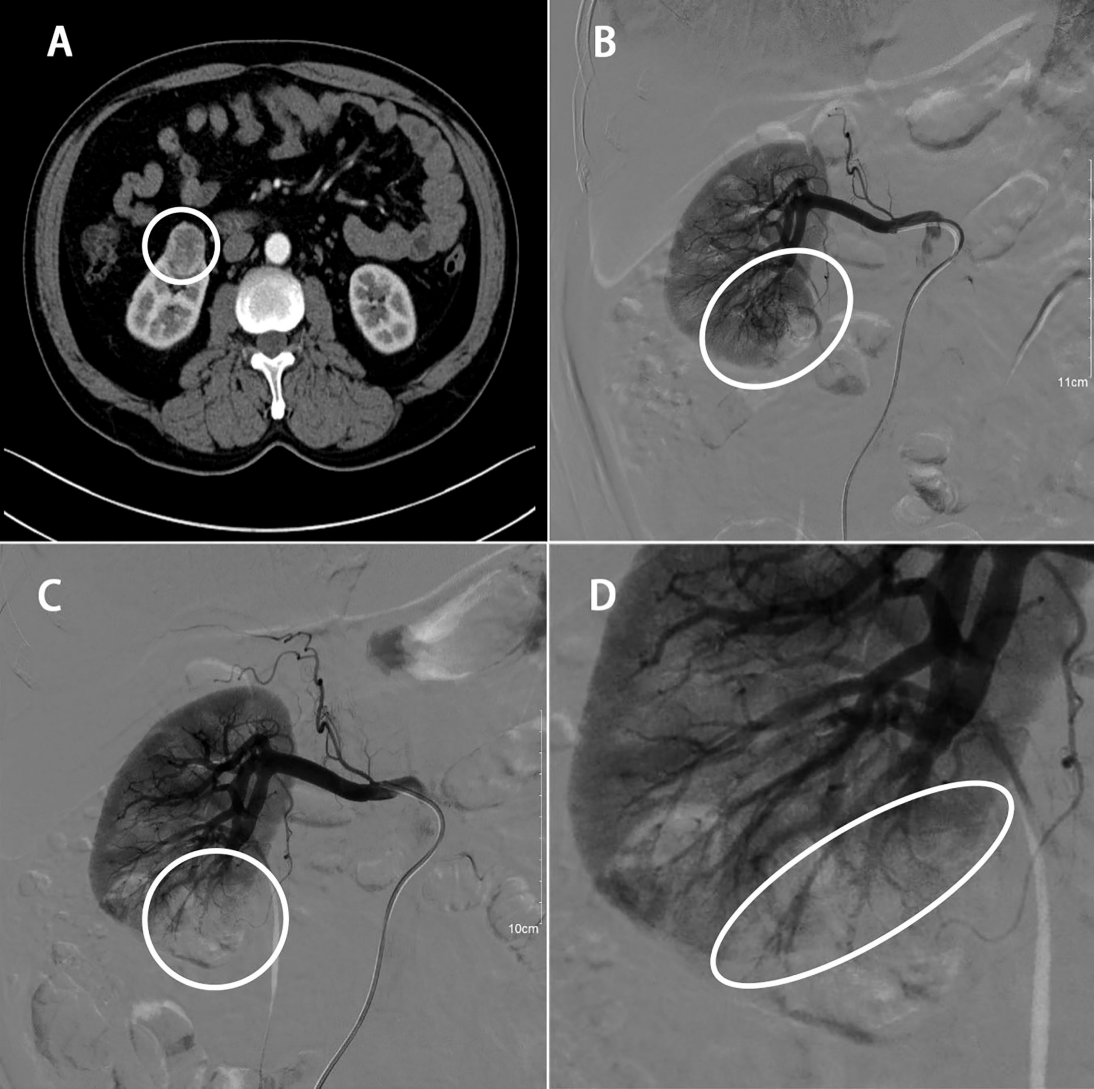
58 years old male with RCC of the right kidney lower pole (40 x 30 x 25 mm). (A) Preoperative enhanced CT scan; (B) renal arteriogram; (C) STE of order artery feeding tumor; (D) final angiogram showing appropriate extent of ischemic area.
